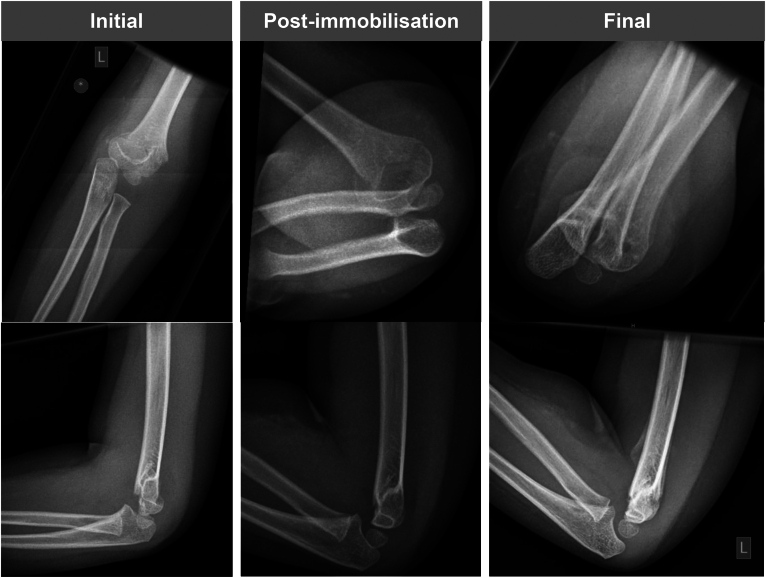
Initial, post-immobilisation and final radiographs
